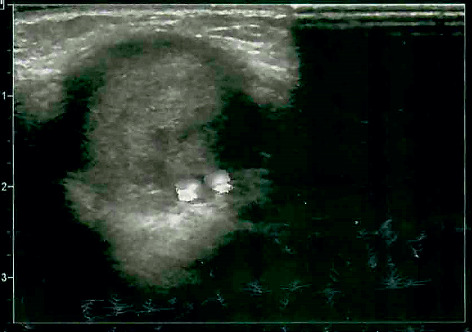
Radial artery aneurysm filled with thrombotic masses (an ultrasound image).
